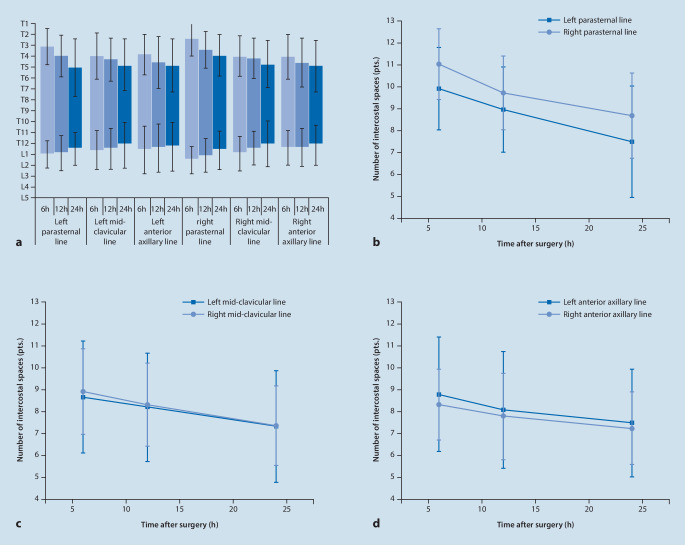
Sensory extent of the erector spine muscle block after surgery. a The extent of the sensory block shown by the vertebral column; b The block extent of parasternal line is shown in number of intercostal spaces; c The block extent of mid-clavicular line is shown in number of intercostal spaces; d The block extent of anterior axillary line is shown in number of intercostal spaces
